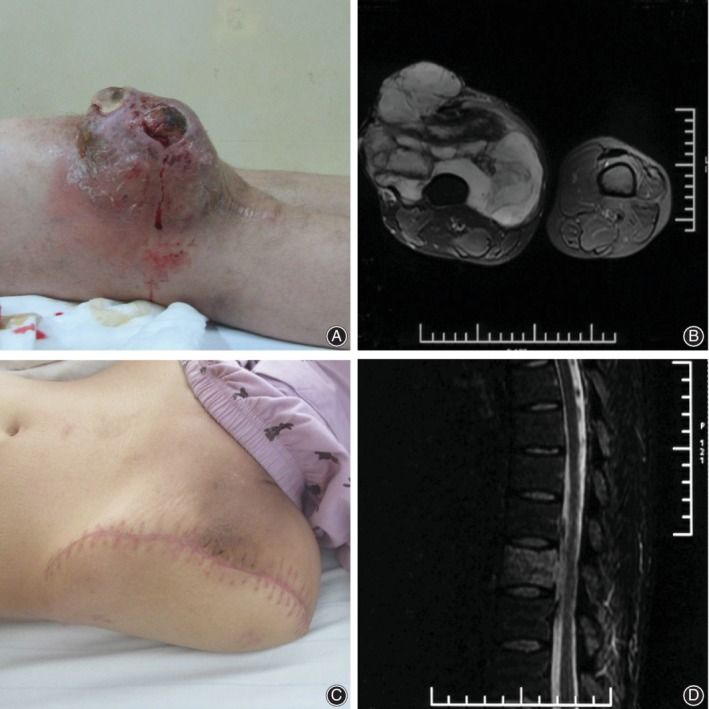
A twenty‐eight years old male patient underwent hip dissection because of myxoid liposarcoma recrudescence. (A) Myxoid liposarcoma recurred at one year after operation. (B) Cross plane MRI showed huge tumor (C) The patient accepted hip dissection. (D) MRI showed the thoracic metastasis of myxoid liposarcoma at three months after hip dissection.
